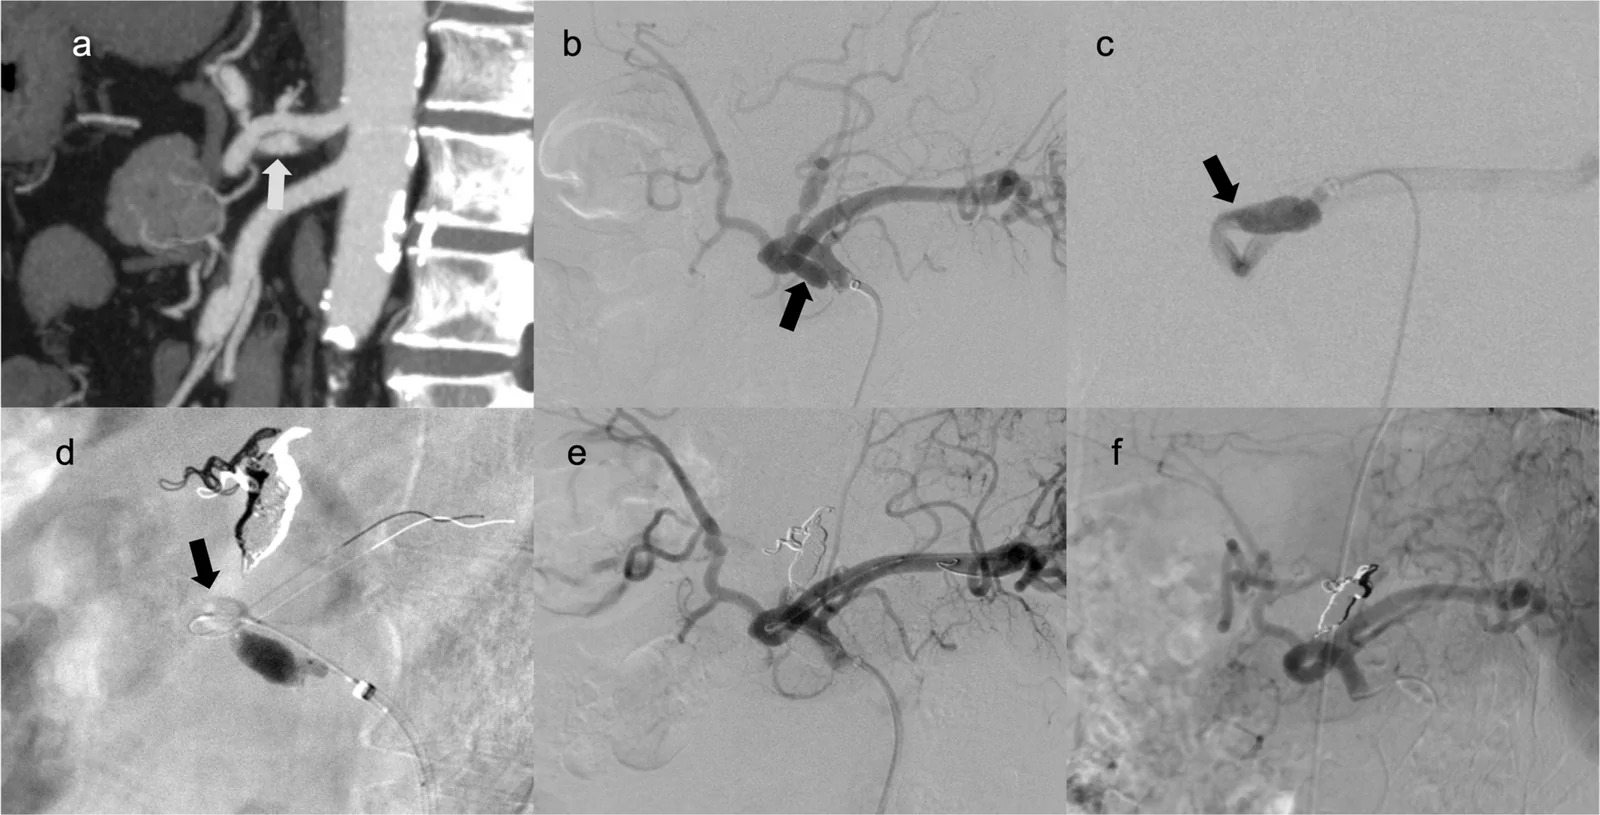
A 55-year-old man with segmental arterial mediolysis (Case 2). a Sagittal reformatted contrast-enhanced computed tomography shows a dissecting aneurysm of the celiac artery (arrow), together with dissecting aneurysms of the left gastric artery and the main trunk of the superior mesenteric artery. b Celiac arteriography shows the false lumen of the dissecting aneurysm (arrow). c Manual contrast injection through a microcatheter positioned in the false lumen shows contrast outflow through the re-entry (arrow) into the splenic artery. d Injection of the N-butyl cyanoacrylate–iodized oil mixture into the false lumen, with a balloon inflated within the true lumen against the re-entry (arrow). A video of the NBCA injection is provided in Supplementary material 1. Coil embolization of the dissecting aneurysm of the left gastric artery had been performed before this procedure. e Celiac arteriography immediately after treatment shows disappearance of false-lumen opacification. f Celiac arteriography 6 years later, obtained during a selective arterial secretin injection test, shows no stenosis of the celiac artery and no residual false lumen
